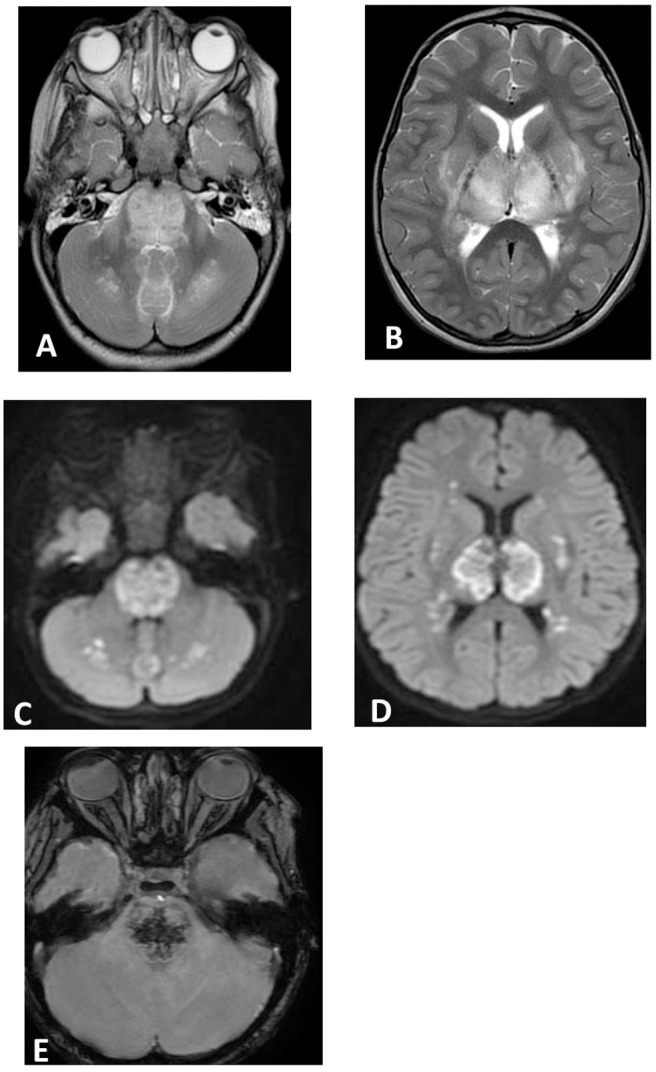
Brain MRI. Axial T2 (A,B), diffusion (C,D), and susceptibility (E) weighted images. There are swelling and abnormal high signal intensity of pons, bilateral cerebellar white matter (A), both thalami, and bilateral putamen (B) with diffusion restriction (C,D) and dark signal intensity with blooming in the pons (E).
